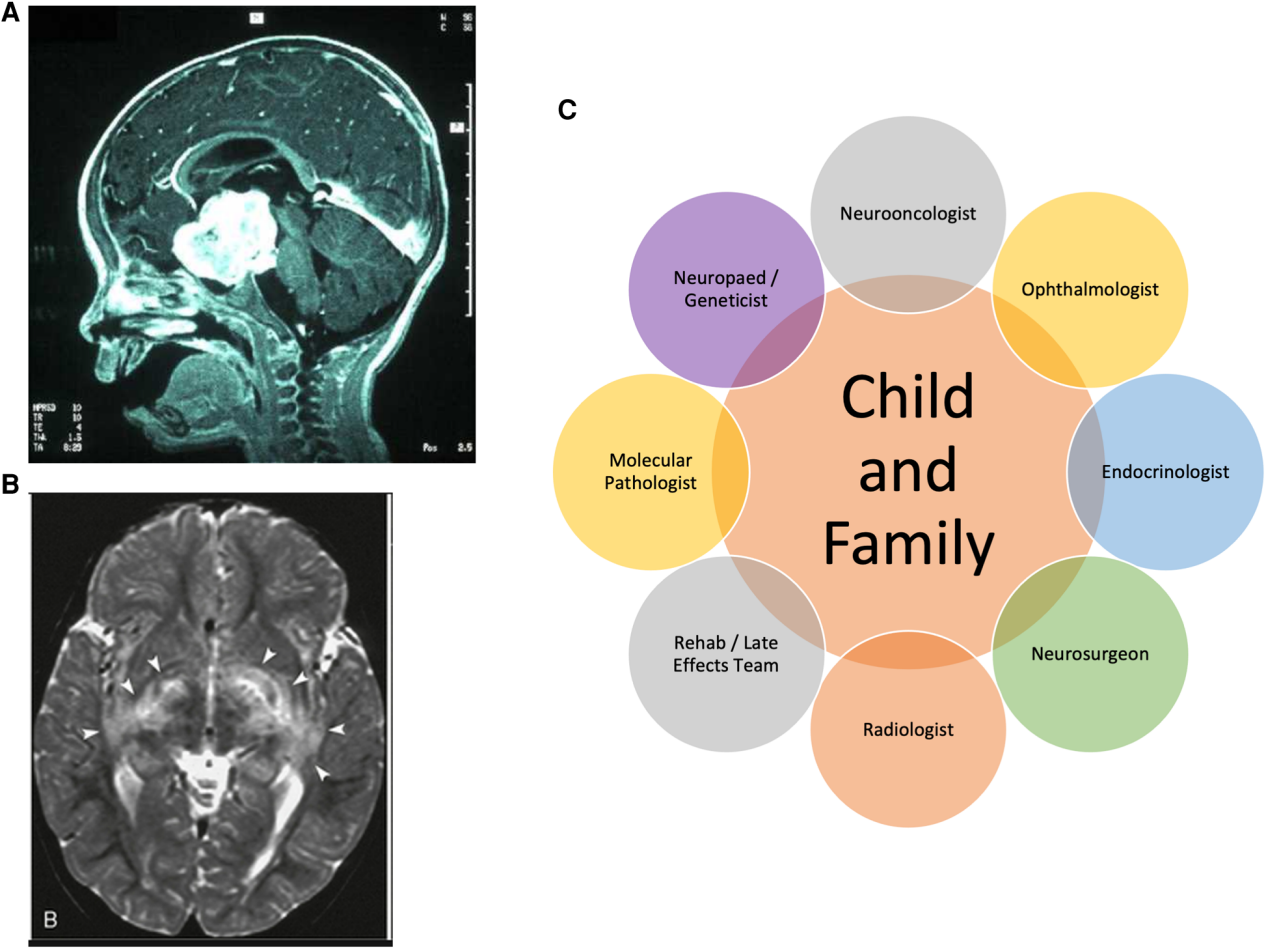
MRI scan of typical (A) sporadic hypothalamic and (B) multi-focal NF1 OPHG involving posterior radiations; (C) clinical specialisms involved in the OPHG multidisciplinary team.
